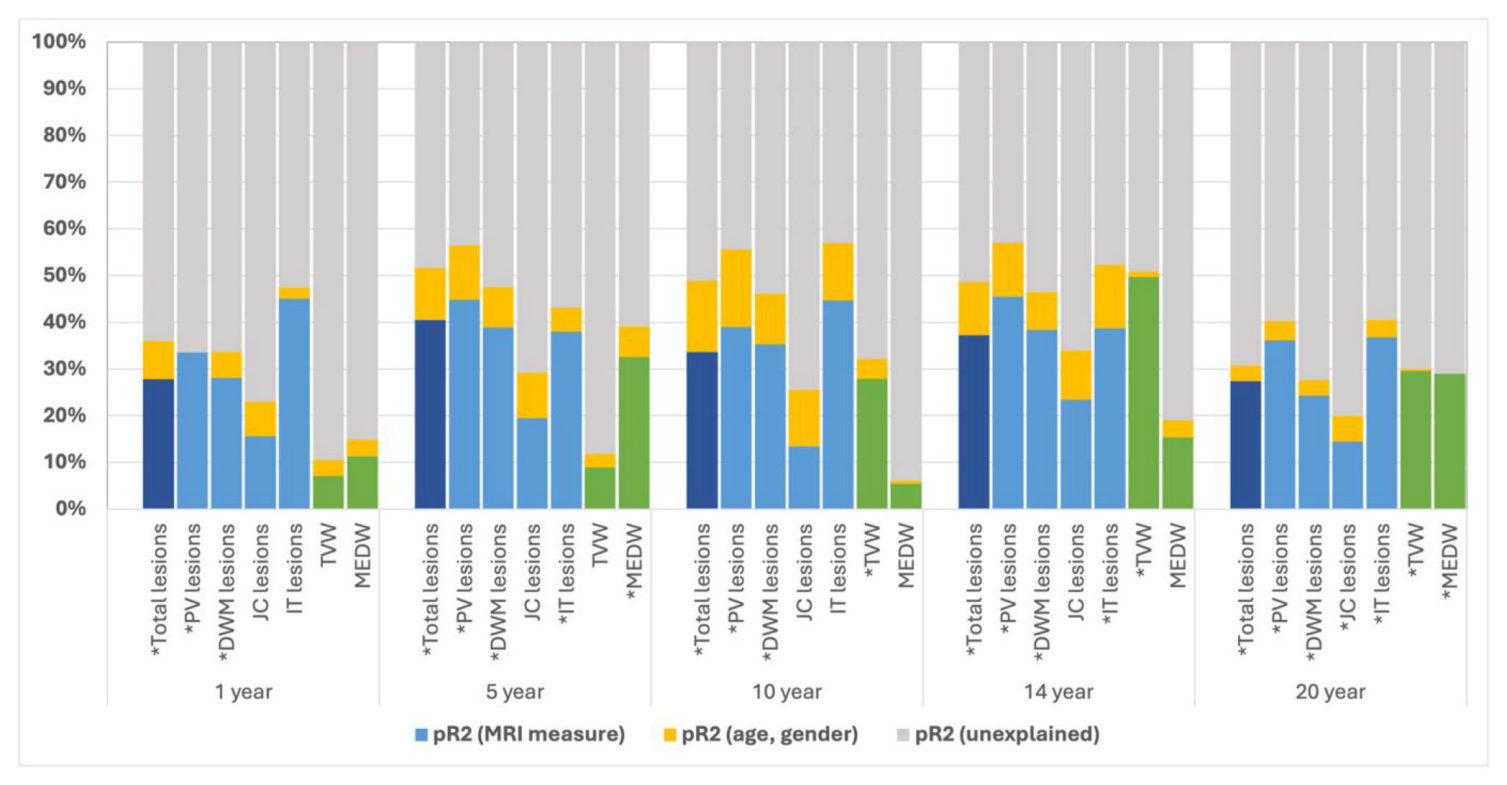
Longitudinal models considering contribution of age, gender and MRI measures (total lesion count, lesion by subtype, linear atrophy measures) towards 30-year SPMS
